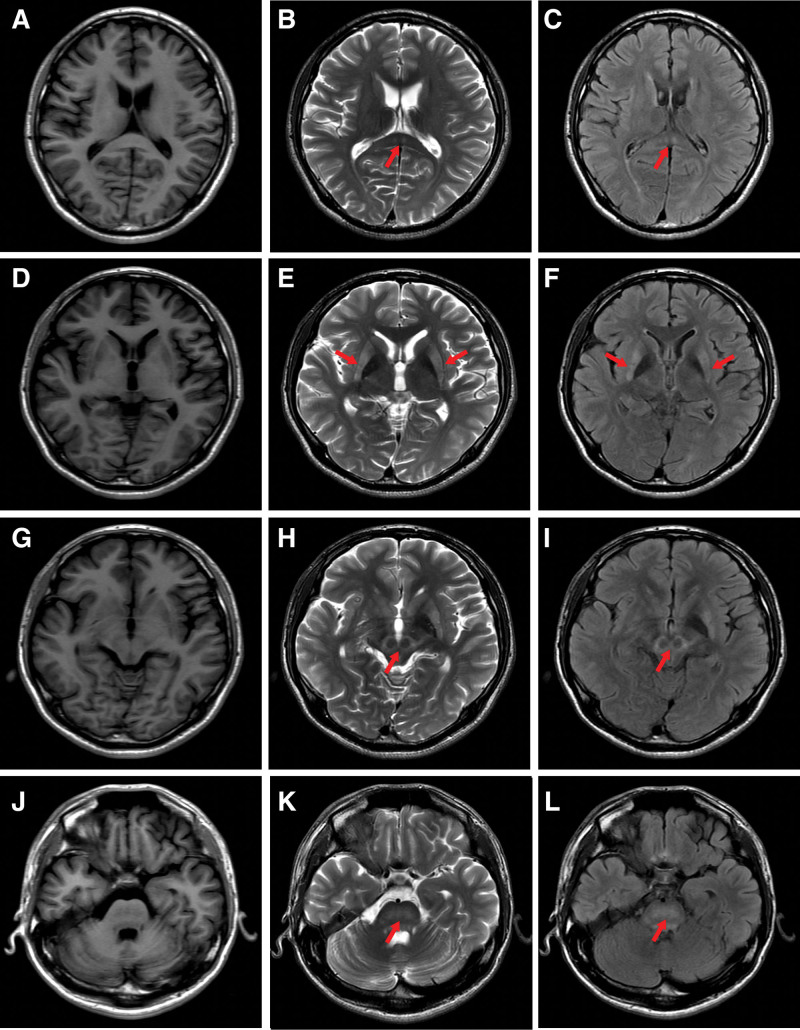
Representative magnetic resonance images of this patient. (A–C) T2-weighted and fluid-attenuated inversion recovery (FLAIR) axial magnetic resonance imaging (MRI) demonstrates hyperintensities in pressure area of corpus callosum (red arrow). (D–F) T2-weighted and FLAIR axial MRI demonstrates hyperintensities in bilateral caudate nucleus and putamen (red arrow). (G–I) T2-weighted and FLAIR axial MRI demonstrates hyperintensities in midbrain, and“face of the giant panda” (red arrow). (J–L) T2-weighted and FLAIR axial MRI demonstrates hyperintensities in pons, and central pontine myelinolysis–like signal changes (red arrow).
